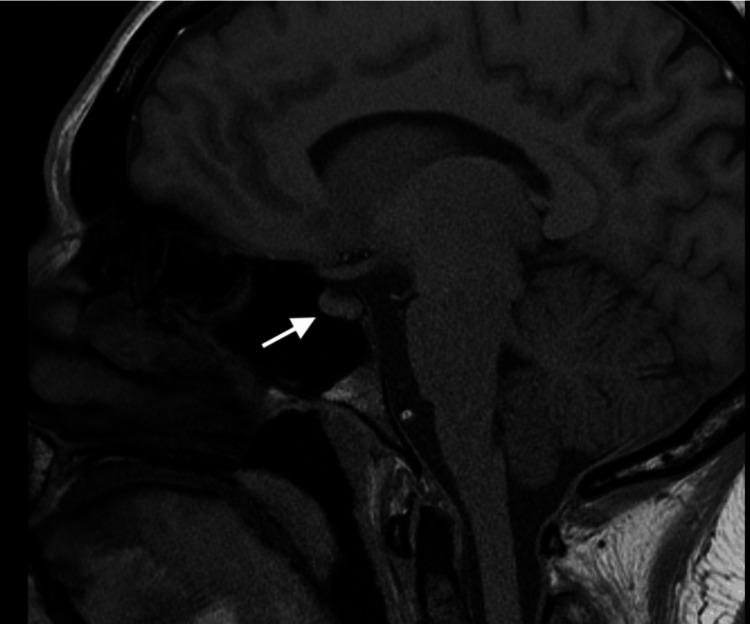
MRI of the brain and pituitary showing stable nodular thickening of the pituitary infundibulum involving the superior portion.
